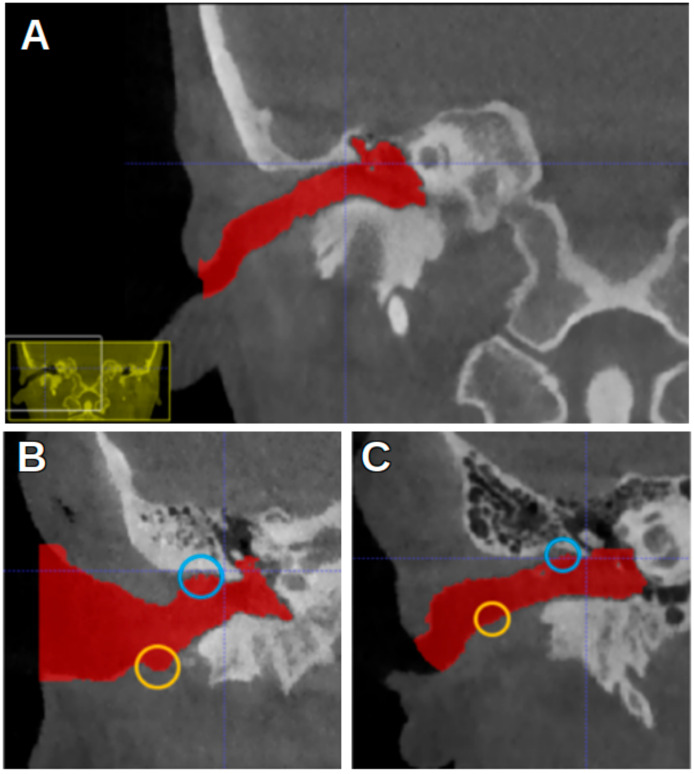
Differences in curvature of EAM. (A) CBCT image overview, from coronal view. (B) Curved EAM. (C) Straight EAM. Blue circles: most superior point of segmentation. Yellow circles: most inferior point.
